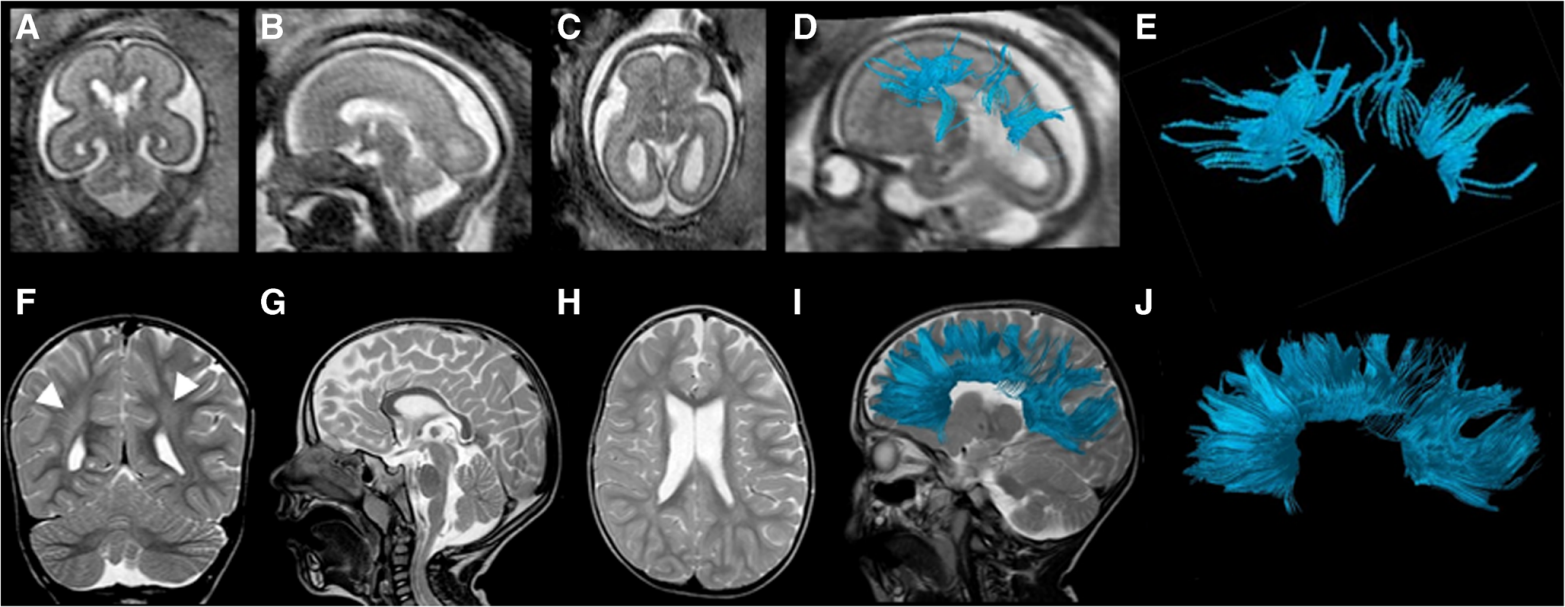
Fetal and postnatal corpus callosum segmentation in a boy (case 2) with periventricular leukomalacia and cortical dysplasia. a–e Age 24+2 gestational weeks. a Coronal T2-weighted (TR/TE 10,653/140 ms, flip angle 90°) MR image. b Sagittal T2-weighted image. c Axial T2-weighted image of the fetal brain. d Overlay of the fiber tracts of the corpus callosum on a sagittal T2-weighted image. e The rostrum and body of the corpus callosum are not well visualized, while the genu and splenium are identifiable on sagittal diffusion tensor imaging (DTI) of the corpus callosum in the fetal brain. f–j Age 2 years. f Coronal T2-weighted (TR/TE 4,000/90 ms, flip angle 90°) MR image of the brain shows asymmetrical, right greater than left, periventricular T2 prolongation (*arrowheads*), compatible with white matter injury. g Sagittal T2-weighted image of the postnatal brain shows an intact corpus callosum. h Axial T2-weighted image. i Segmented sagittal view of the callosal fiber tracts overlaid on a sagittal T2-weighted image of the postnatal brain appears fully intact. j The rostrum, genu, body and splenium are identifiable on this sagittal DTI of the corpus callosum segmentation. *TE* echo time, *TR* repetition time
